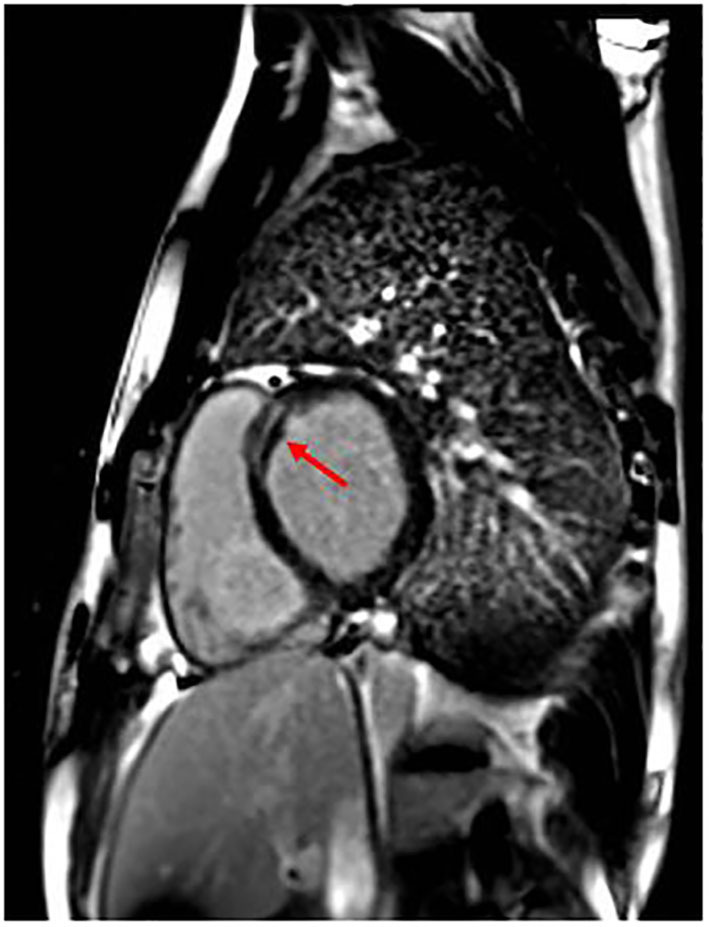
Patient 1 focal image of the cardiac magnetic resonance imaging (MRI) in short-axis view showing LGE at the intramyocardial wall at the medium–basal level of the interventricular septum. The red arrow indicates the areas with major involvement of LGE (late gadolinium enhancement).
